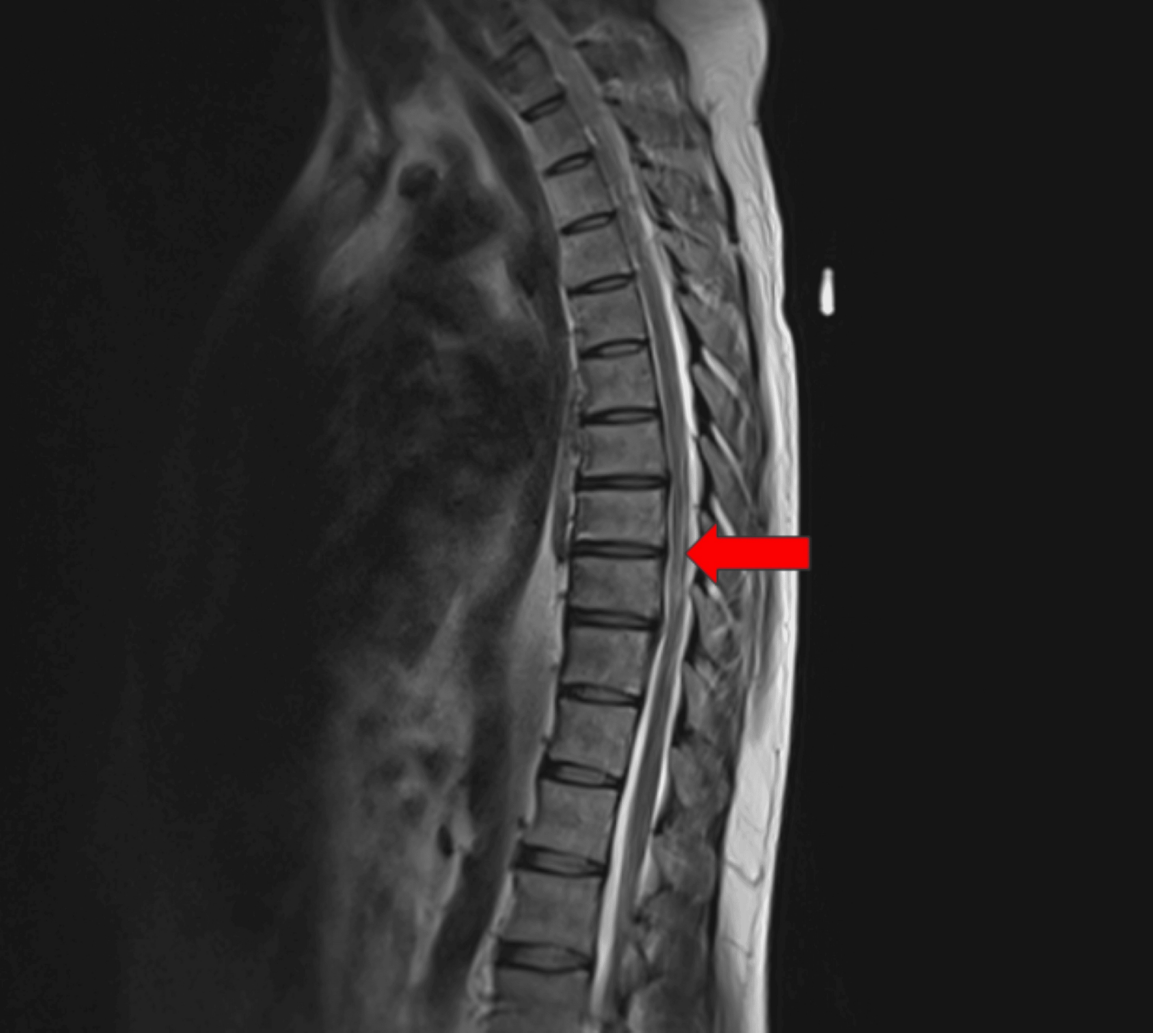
Sagittal view of cord signal abnormalities confined to the dorsal columns is most consistent with selective degeneration secondary to vitamin B12 deficiency. The red arrow indicates the areas of subacute combined degeneration on MRI.
